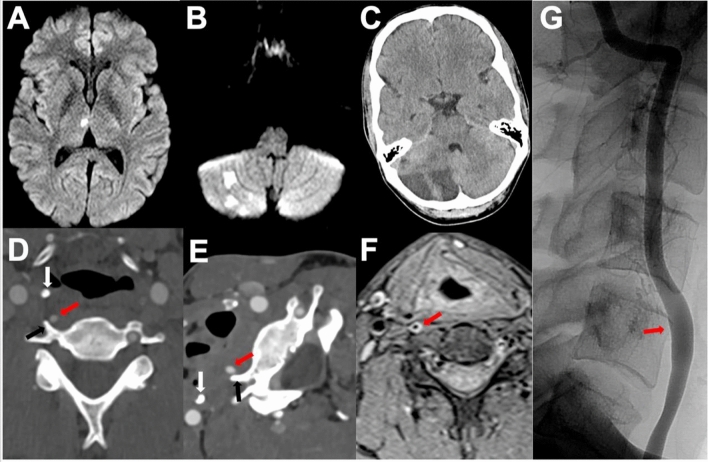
(A, B) Diffusion-weighted sequences depict right thalamic infarction (A) and right PICA infarcts (B) at d0. C Right-sided SCA infarct was observed in computed tomography on d118. No luminal irregularities were detected in aVA-C4 (red arrow) at d15 without (D) and with neck rotation to the right (E). F Fat-saturated T1w-sequences are depicted at d40. T1w hyperintensity of aVA-C4 is labeled with a red arrow. G DSA of aVA-C4 depicts minimal dilatation of aVA-C4 proximal to the entrance in the foramen transversarium C4 at d38
